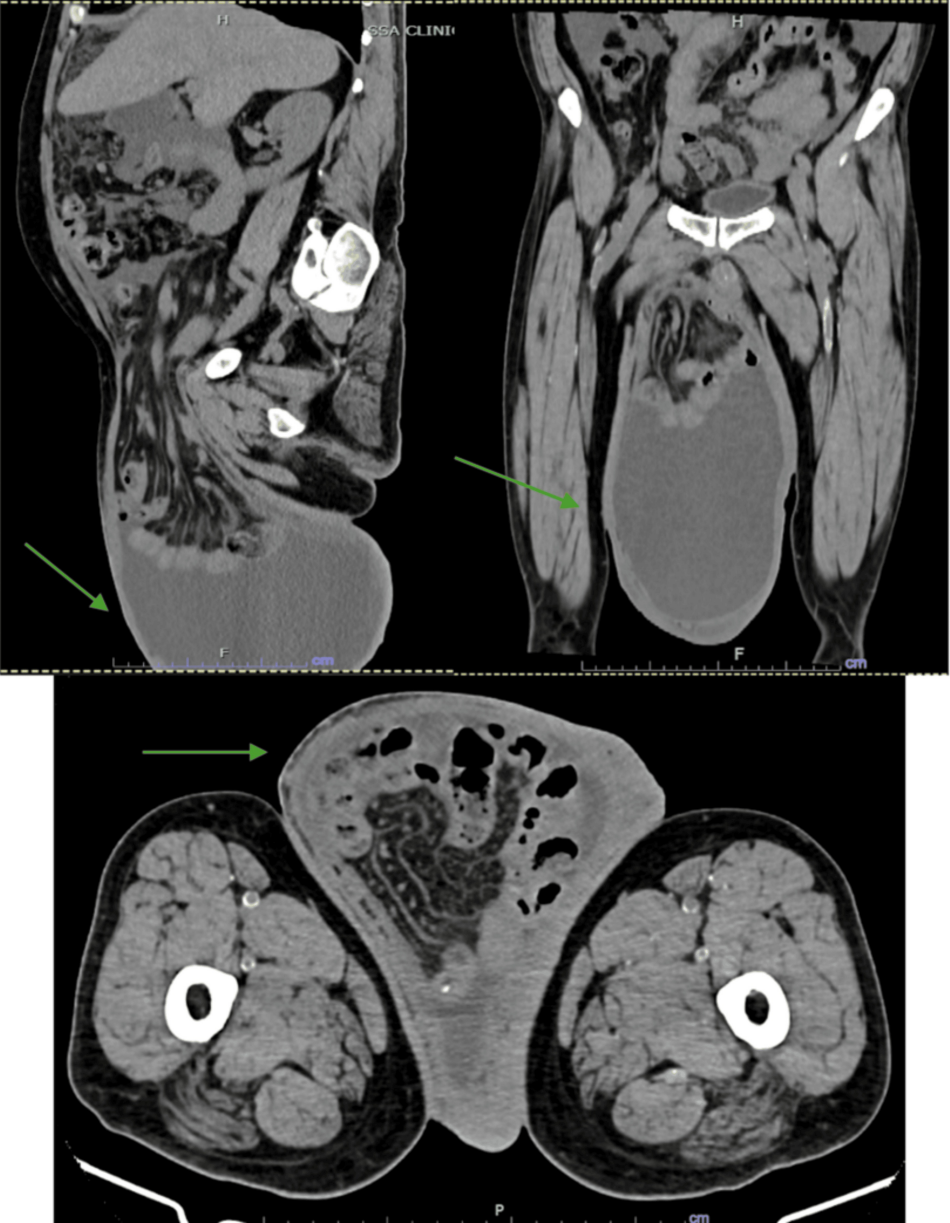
CT scan showing the location of the inguinoscrotal hernia
